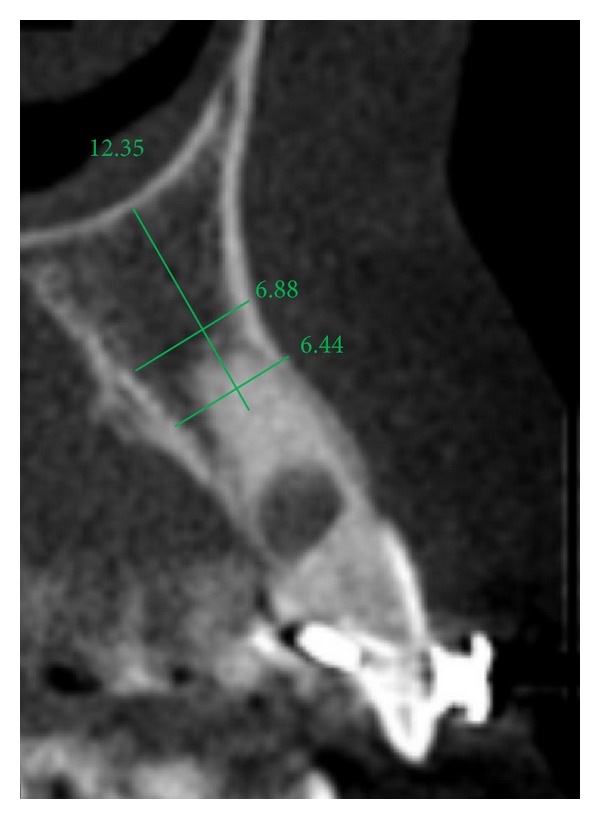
Initial computed tomography.
